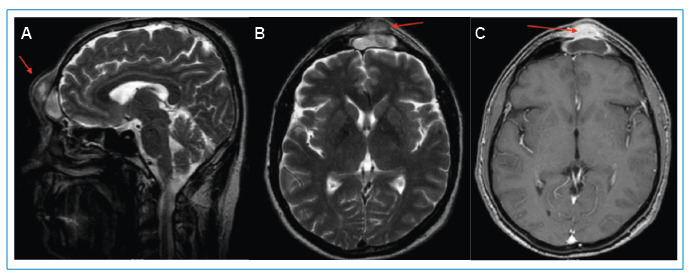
Pott’s puffy tumor in the frontal sinus (arrows) on the sagittal (A) and axial (B,C) cranial MRI scans.
